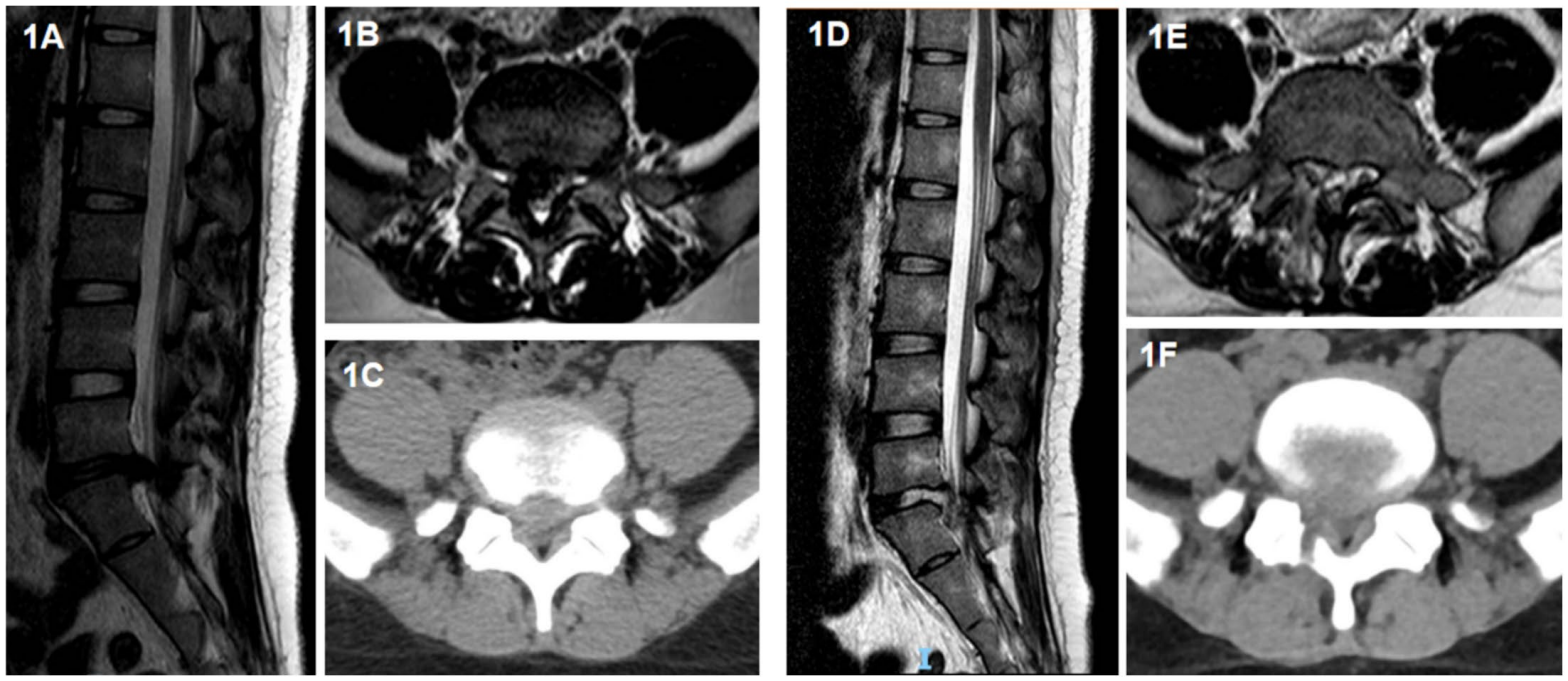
A 35-year-old female patient with right-sided protrusion of the L5/S1 intervertebral disc who underwent PEID surgery. (A) Sagittal view of preoperative lumbar magnetic resonance imaging (MRI); (B) horizontal view of preoperative lumbar MRI; (C) horizontal view of preoperative lumbar computed tomography (CT); (D) sagittal view of postoperative lumbar MRI; (E) horizontal view of postoperative lumbar MRI; (F) horizontal view of postoperative lumbar CT.
